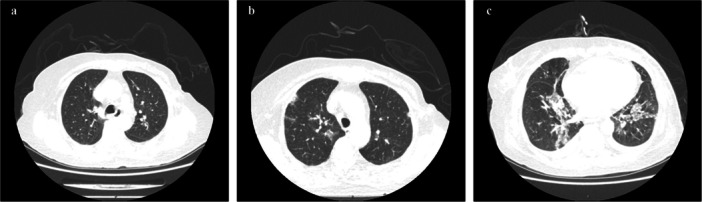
CT scan of the chest. (a) CT scan of the chest showed normal lung. (b) High‐resolution CT of the chest showed multiple ground‐glass density lesions in both lungs after treatment of T‐DXd. (c) High‐resolution CT of the chest showed more and larger ground‐glass density lesions and new sheet‐like consolidation lesions, accompanied by interlobular septal thickening.
